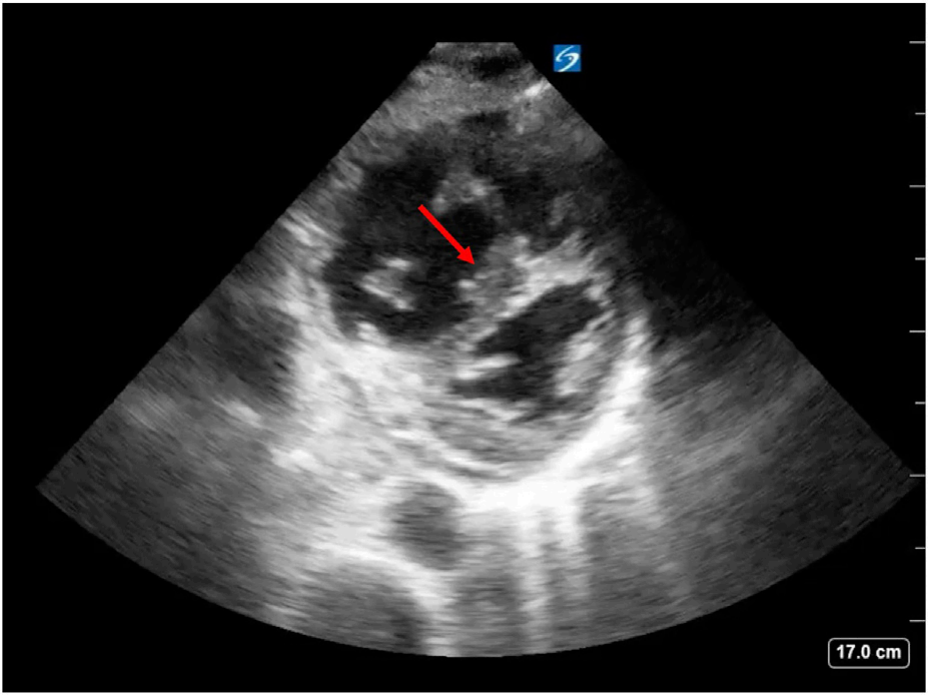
Negative swings in ITP, as seen with spontaneous inspiration in patients with COPD exacerbation, lead to increased RV end-diastolic volume. This effect in combination with increased RV afterload can cause RV dilation and intraventricular septal shift towards the LV leading to decreased LV end-diastolic compliance, decreased LV end-diastolic volume and decreased cardiac output. This is known as “D sign” on echocardiography (arrow). Image source: Dr. Christopher Schott, University of Pittsburgh Medical Center.
